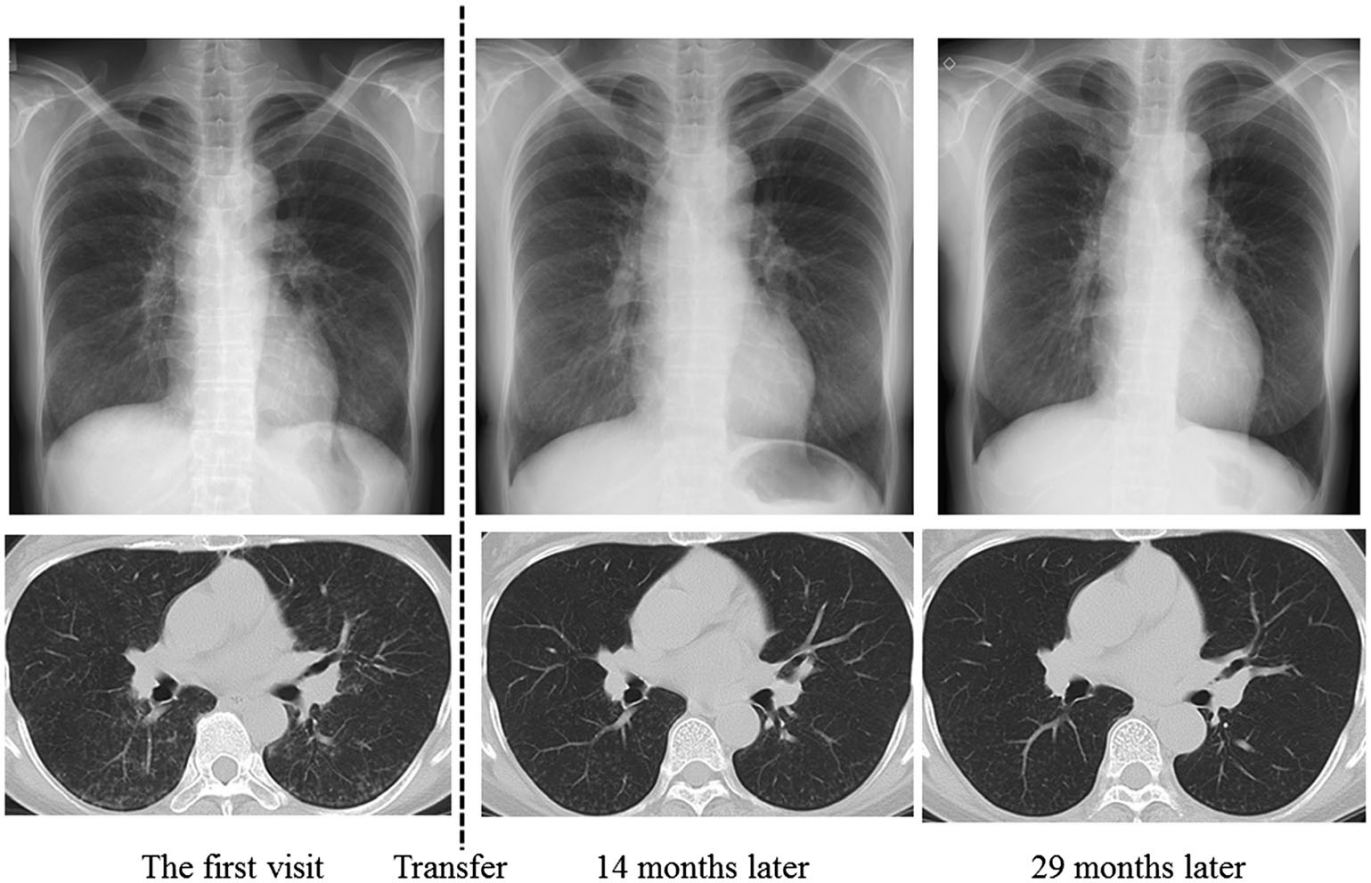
Chest X‐rays and high‐resolution computed tomography images on the first visit showed diffuse bilateral ground‐glass opacities and poorly defined centrilobular nodules. At 14 and 29 months after transfer to a different department of the factory, the images showed marked improvement.
